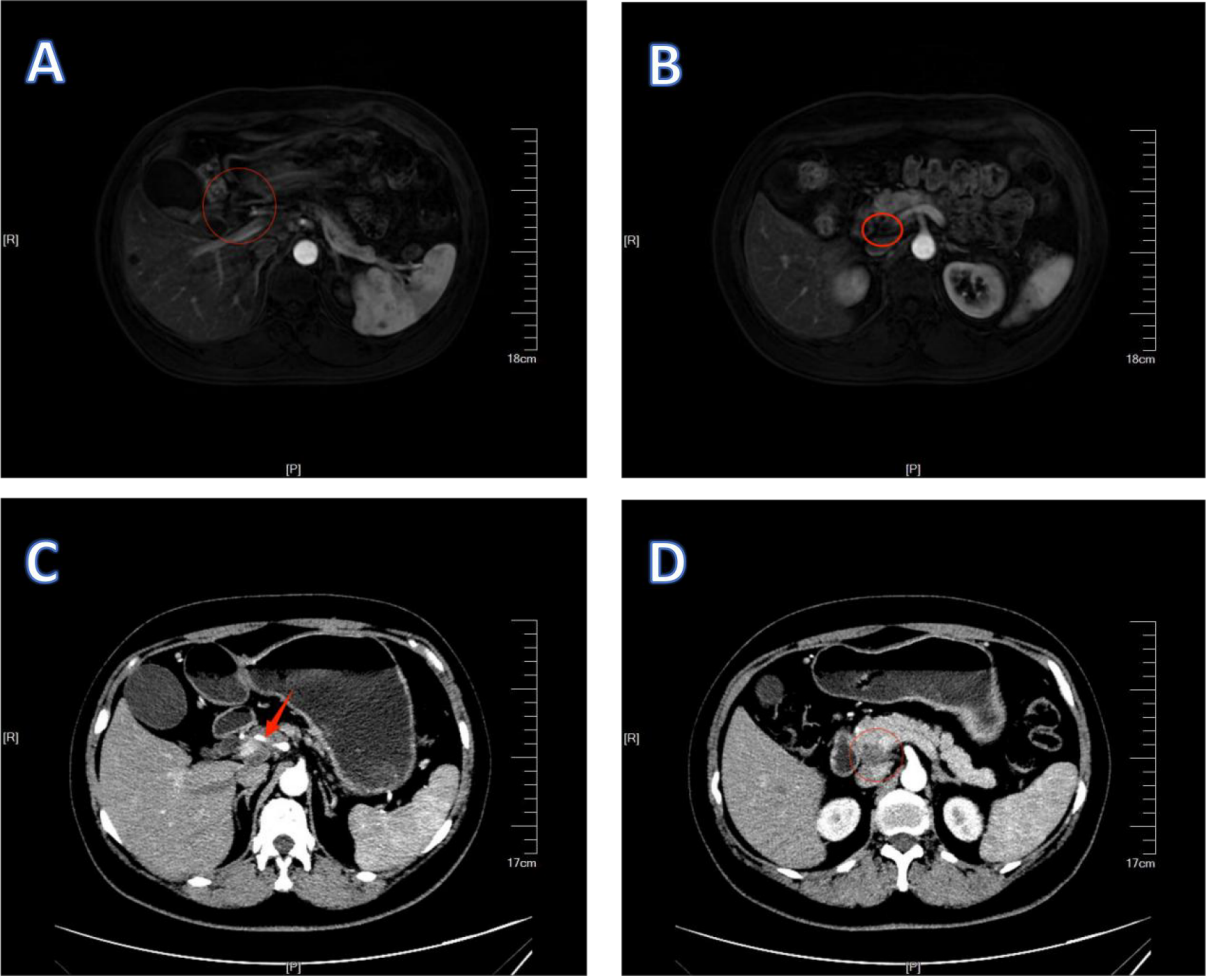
1Preoperative examination of patient 1. (A, B) are MRI images, showing that the lymph nodes have shrunk. (C, D) are CT images. (C) shows an improvement in the condition where the lymph nodes surround the hepatic artery.
